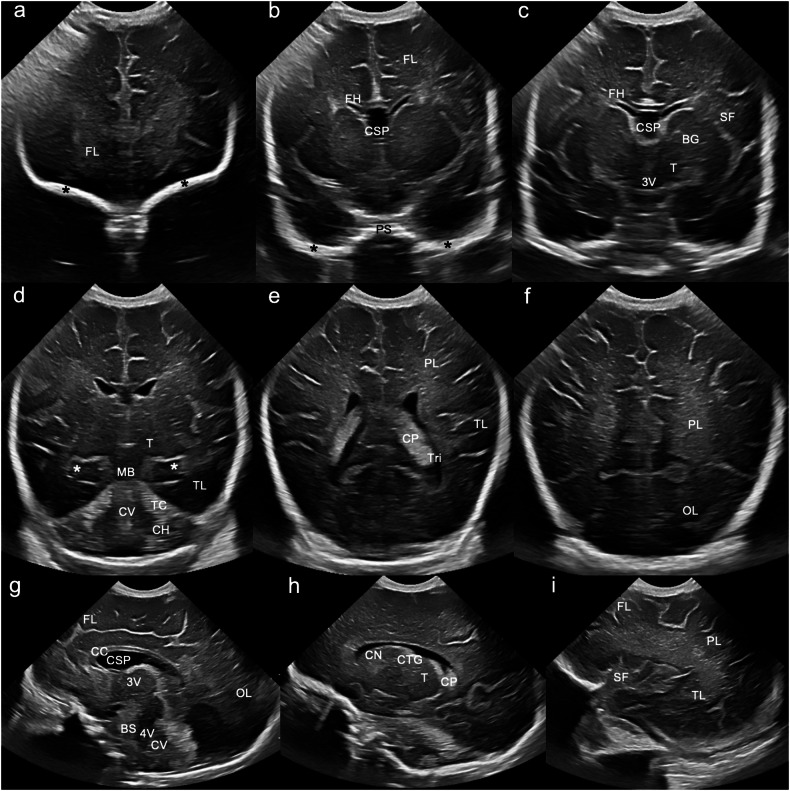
Standard sonographic views through the anterior fontanelle. Six coronal and five sagittal images are acquired: a The first coronal image depicts the frontal lobes (FL). The bony orbital roofs (*) in the far field act as a landmark. Note the hypoechoic grey matter peripherally and the hyperechoic subcortical and deep white matter centrally. b The second coronal image depicts the frontal lobes and frontal horns of the lateral ventricles (FH). Note the cavum septum pellucidum (CSP) interposed between the two frontal horns. The greater wings of sphenoid (*) and planum sphenoidale (PS) are visible in the far field. c The third coronal image is at the level of the third ventricle (3V) and includes the frontal horns of the lateral ventricles (FH), basal ganglia (BG)/thalami (T) and Sylvian fissures (SF). d The fourth coronal image is at the level of the cerebellum, and depicts the temporal lobes (TL), thalami (T), midbrain (MB), tentorium cerebelli (TC), cerebellar vermis (CV), and cerebellar hemispheres (CH). The far-field “C shape” of the parahippocampi and medial temporal lobes (*) serves as a landmark. e The fifth coronal image depicts the parietal (PL) and temporal lobes (TL) at the level of the lateral ventricular trigones (Tri). Note the echogenic choroid plexus (CP) within the lateral ventricular bodies. f The sixth and final coronal image depicts the parietal (PL) and occipital lobes (OL). g The first sagittal image depicts the midline structures—the corpus callosum (CC), cavum septum pellucidum (CSP), third (3V) and fourth ventricles (4V), brainstem, and cerebellar vermis (CV). Note the slightly increased echogenicity of the third and fourth ventricles compared to the CSP due to partial volume averaging. h The second sagittal image is the first parasagittal view, showing the “C-shaped” lateral ventricle, with the caudothalamic groove (CTG) separating the caudate nucleus (CN) anteriorly from the thalamus (T) posteriorly. Note the echogenic choroid plexus within the lateral ventricular body. i The third sagittal image is the second parasagittal image at the level of the Sylvian fissure (SF), depicting the frontal (FL), parietal (PL) and temporal (TL) lobes. h and i are repeated on the contralateral side to complete the sagittal imaging assessment
